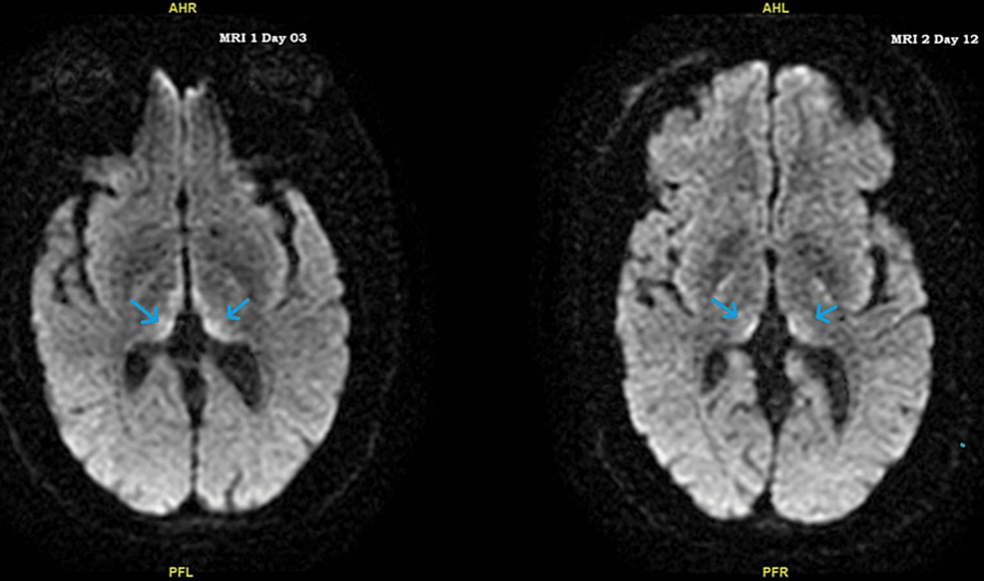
Comparison of MRI brain without contrast on day 03 and day 12. The first MRI shows diffusion-weighted imaging (DWI) showing typical Wernicke's encephalopathy changes in the bilateral medial thalami compared to the second MRI showing improved thalami. Blue arrows show a limbic system
